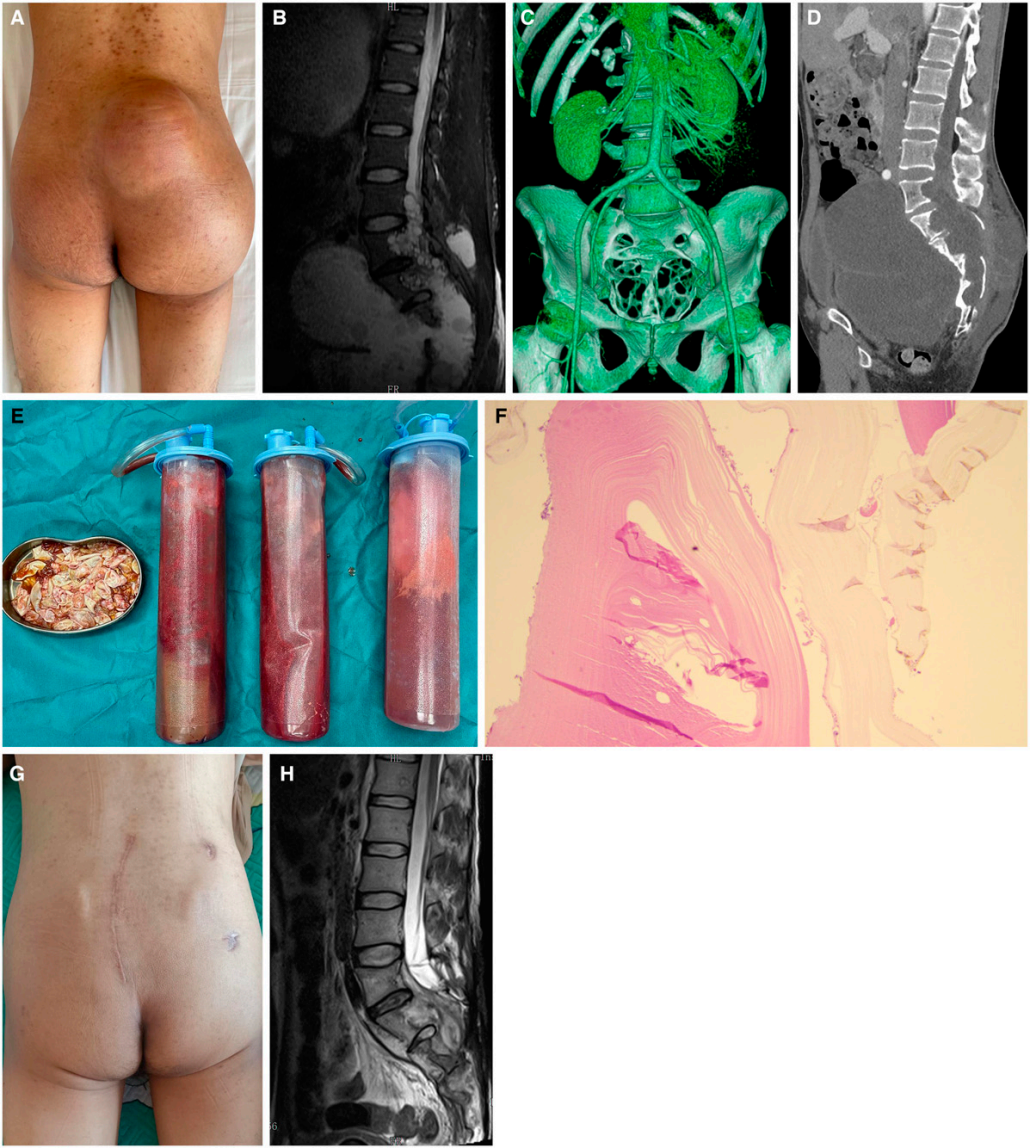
(A) Preoperative body photograph. (B–D) Preoperative magnetic resonance imaging (MRI) and computed tomography scans showed expansile multicystic lesions and destruction of the L5 vertebra, sacrum, and coccyx. (E) Removed lesions. (F) Lamellar membranous structure confirmed the diagnosis of cystic echinococcosis (×100). (G and H) Body photograph and MRI at 5-month follow-up.
